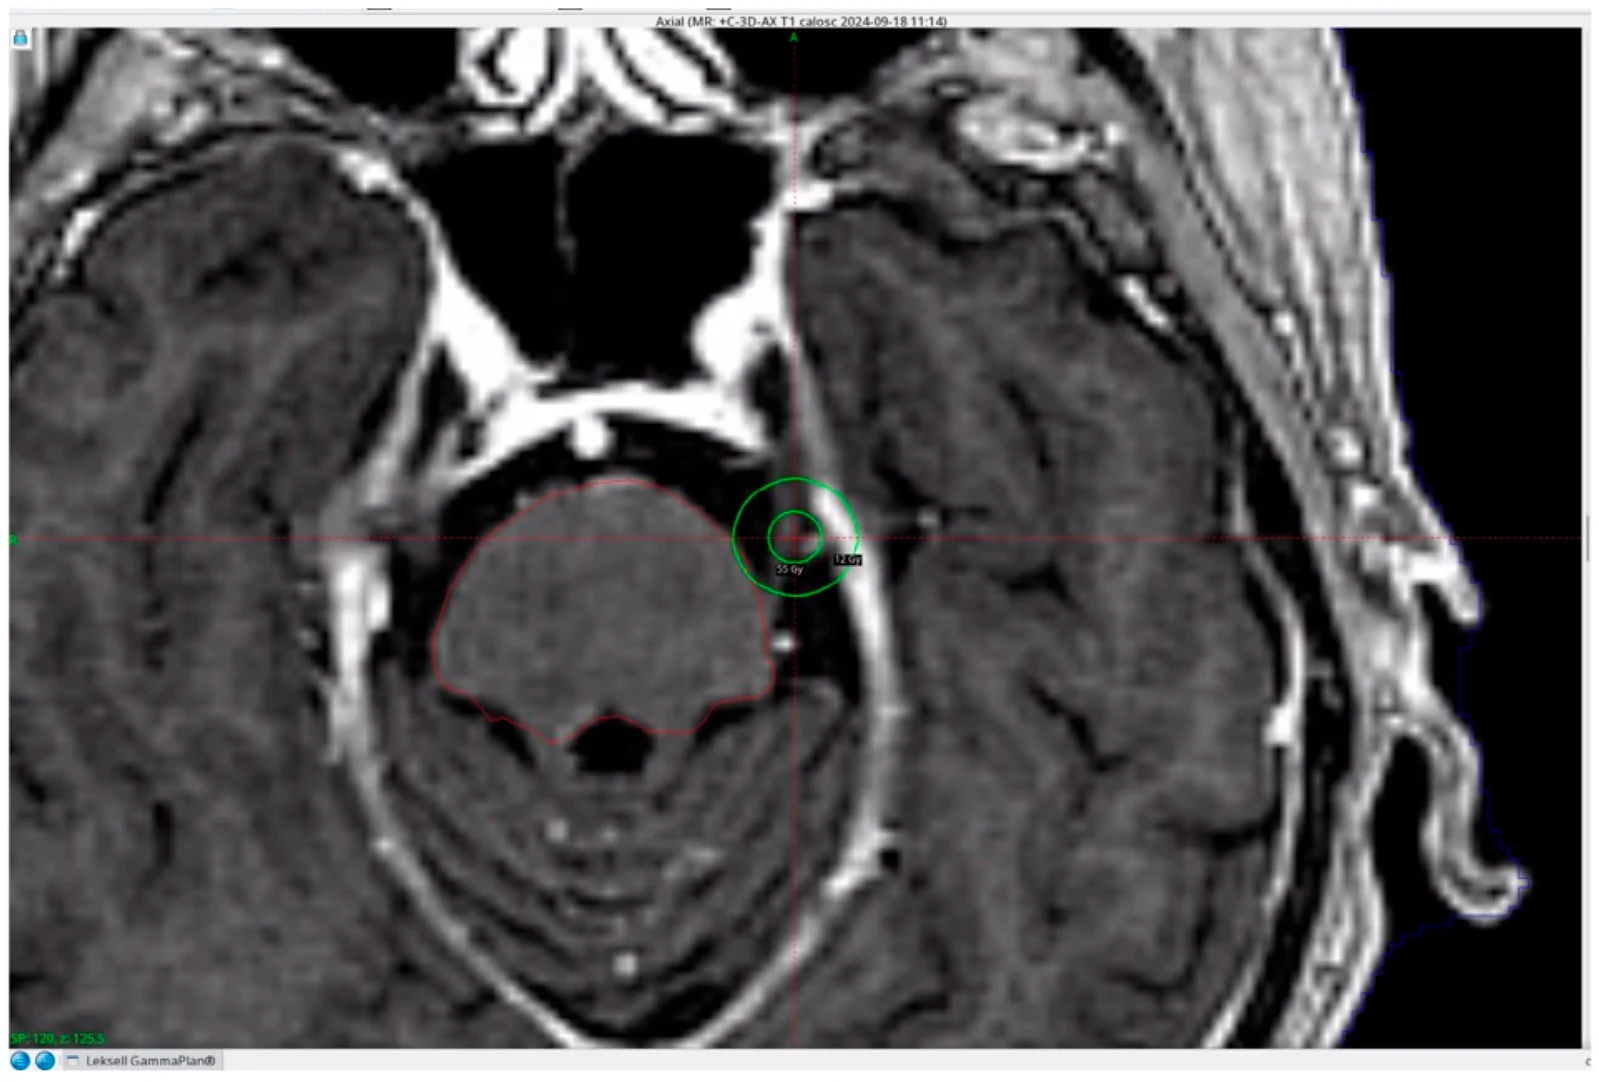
Contrast-enhanced axial T1-weighted magnetic resonance image showing a single 4-mm isocentre applied to the cisternal portion of the left trigeminal nerve. The conflicting vessel is located in the vicinity of the prescribed 85 Gy isodose.
